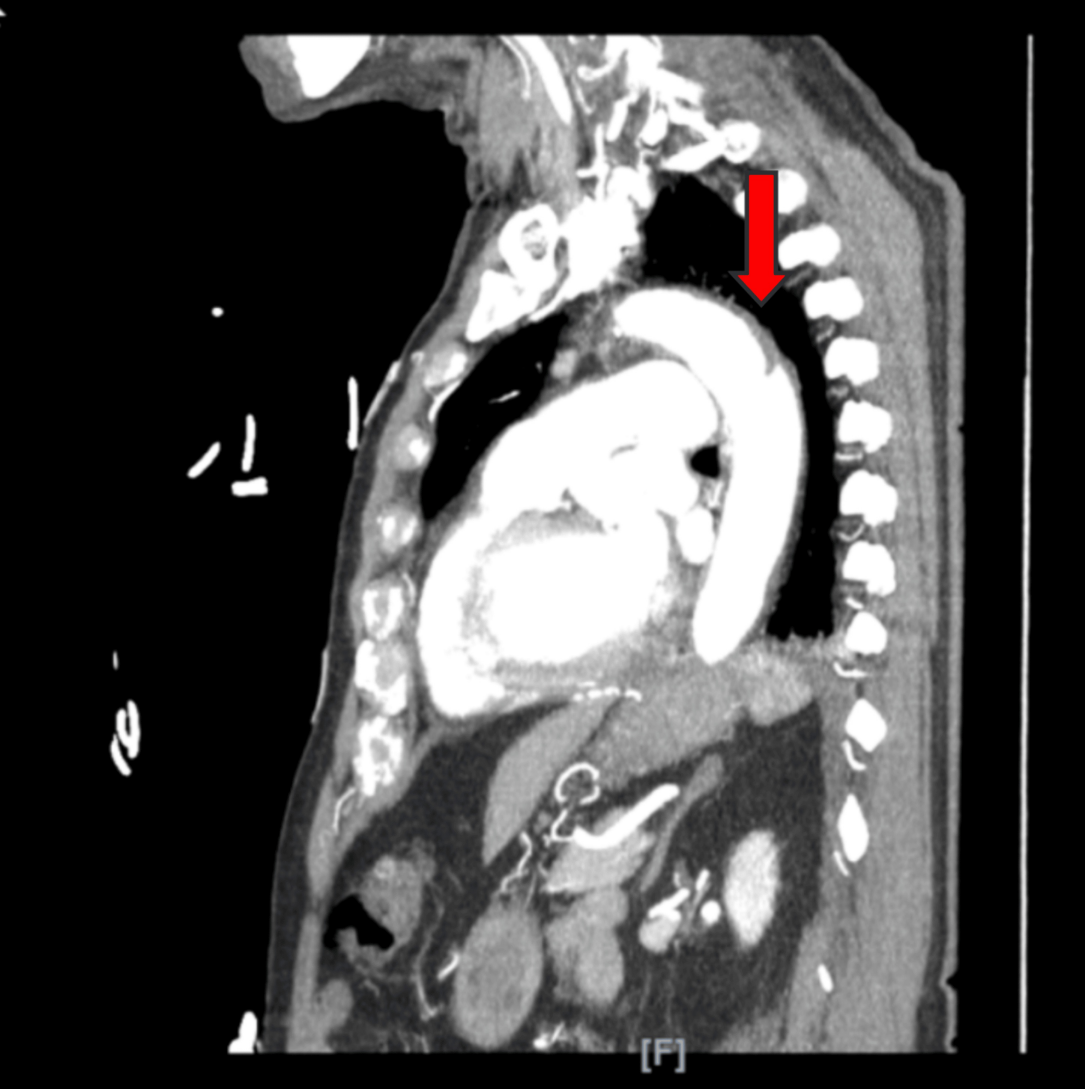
Sagittal CT cut revealing the descending aortic defect CT scan revealing AAD of the descending aorta AAD: Acute aortic dissection
